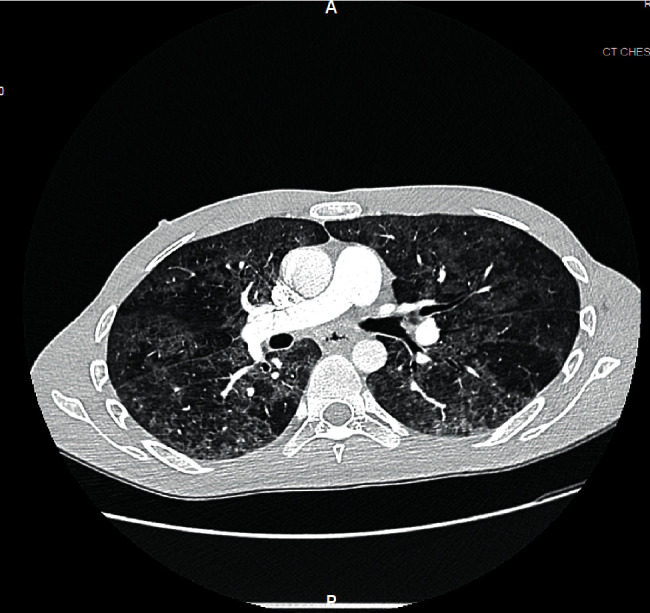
Chest CT showing diffuse bilateral ground glass opacities.
